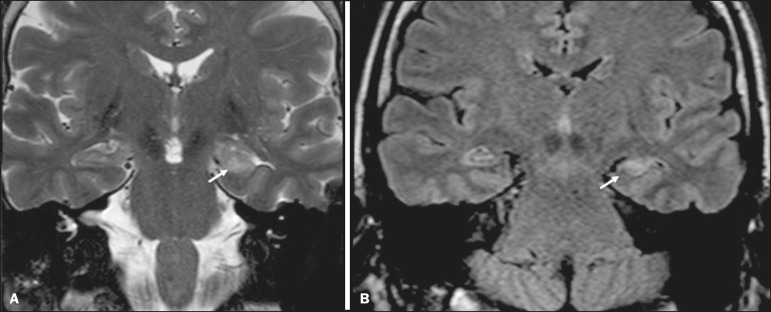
Limbic encephalitis. Coronal T2 sequence (A) and FLAIR sequence (B), showing a hyperintense signal in the left hippocampus.
